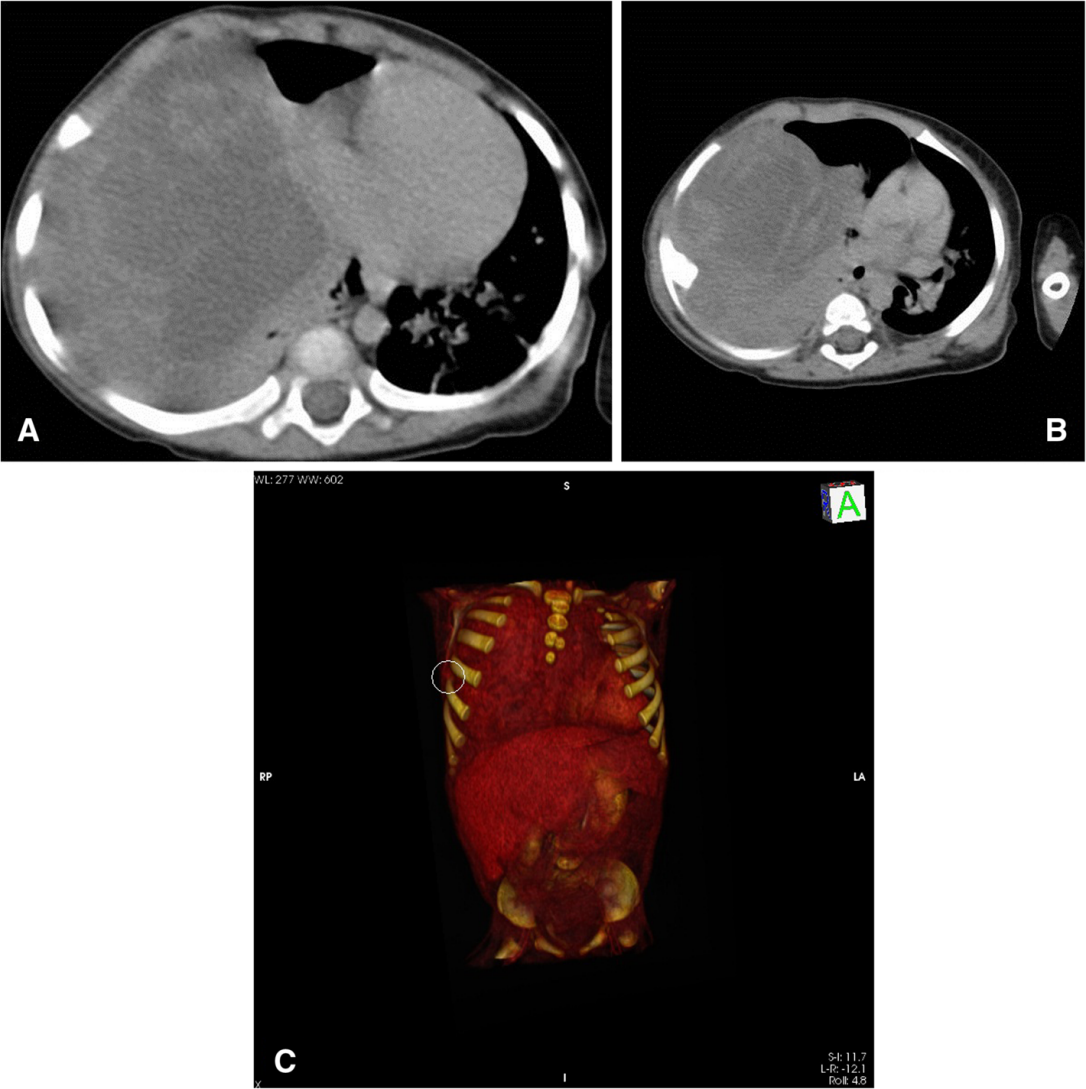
Computed tomography scanning of the thorax. Demonstrating the presence of an enormous mass, causing a tracheal displacement with complete collapse of the lung parenchyma (A) with structural alteration in the 6th right coastal arc, along the midaxillary line (B) better demonstrated by the three-dimensional reconstruction (C).
